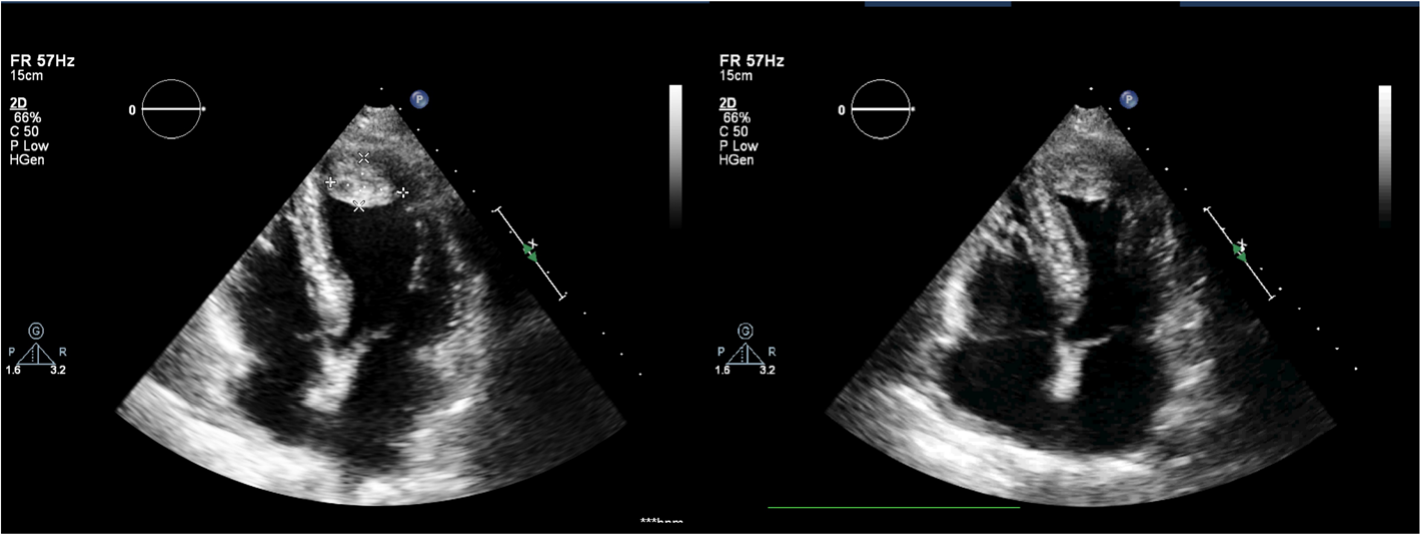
Follow up transthoracic echocardiogram, done 1 week later, showing only mild hypokinesia of the apex of the *left* ventricle and the thrombus which had reduced in size (2.1 cm × 1.8 cm)
